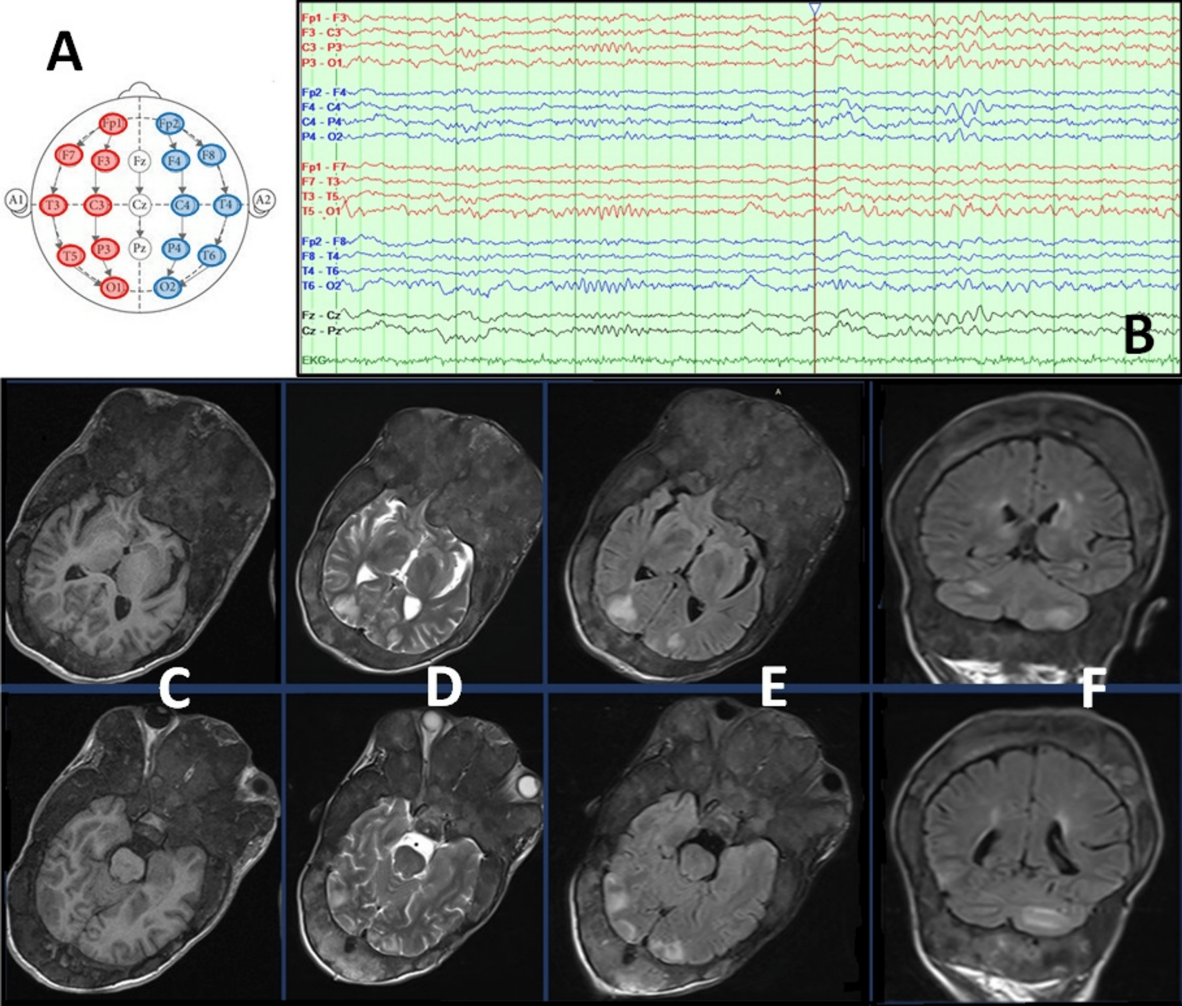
Electroencephalography and brain imaging (A) The International 10–20 system was followed for the placement of scalp electrodes during the EEG exam. (B) No epileptiform activity was observed. Note the predominance of low-frequency, high-amplitude (delta and theta) waves, primarily corresponding to the posterior scalp electrodes, indicating reduced neural activity. No lateralization was observed. (C-E) Axial brain MRI images: T1-weighted (C), T2-weighted (D), and T2-weighted cerebrospinal fluid-suppressed (FLAIR) (E) at the level of the interthalamic adhesion (top) and the interpeduncular cistern (bottom). Areas of hypointense T1 and hyperintense T2 signals are evident in the parieto-occipital areas bilaterally, along with compression of the optic nerves by fibro-osseous tissue bilaterally. (F) Coronal FLAIR brain MRI at the level of the cerebellar vermis and vein of Galen (top) and the posterior part of the straight sinus near the confluence of sinuses (bottom). Areas of hyperintense T2 signals are observed in the cerebellar hemispheres bilaterally, as well as in the centrum semiovale and corona radiata. EEG: electroencephalogram, MRI: magnetic resonance imaging, FLAIR: fluid-attenuated inversion recovery
